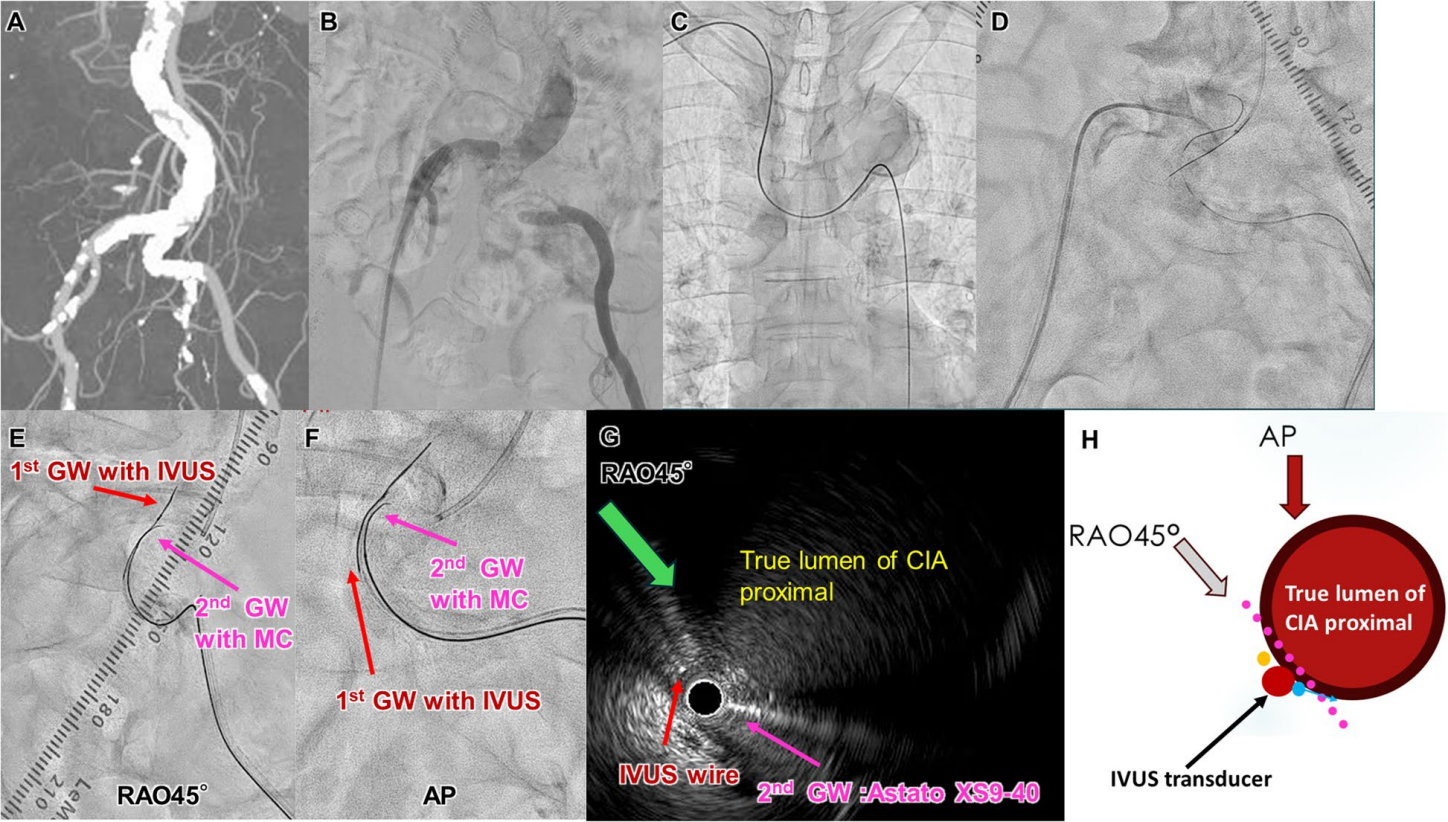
A Contrast-enhanced computed tomography and control angiography showed severe stenosis of the right CIA and occlusion of the left CIA. C, D Antegrade wiring was very difficult because of the tortuous descending aorta and left CIA. E, F Fluoroscopic images of right anterior oblique 45° and anteroposterior view, respectively (red arrow: first GW with IVUS, pink arrow: second GW with MC). G, H IVUS findings of proximal CIA. The IVUS transducer and second GW were in the SS (red arrow: IVUS on the wire, pink arrow: second GW). The green arrow indicates the right anterior oblique 45° view and the red arrow indicates the anteroposterior view
